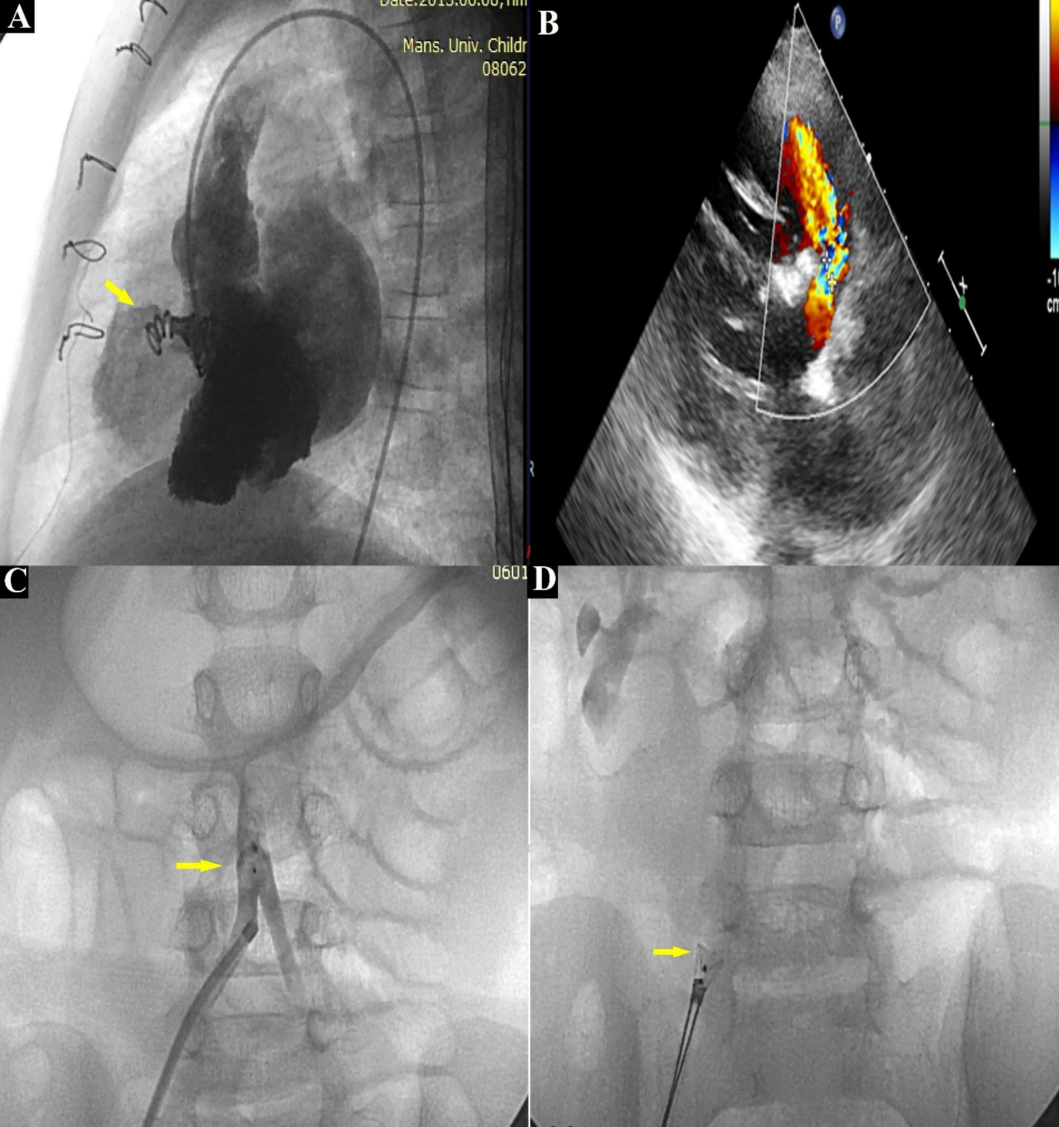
Early complications of VSD device closure (A) LV angiography demonstrating coil in place across subaortic perimembranous VSD with mild residual shunt (B) Post-procedure transthoracic echocardiography showing residual shunt (C) Hand injection in right common iliac artery demonstrating embolized ADO I into the bifurcation of the aorta without causing obstruction (D) ADO I embolized Device was successfully snared. Yellow Arrow is pointing at the device
